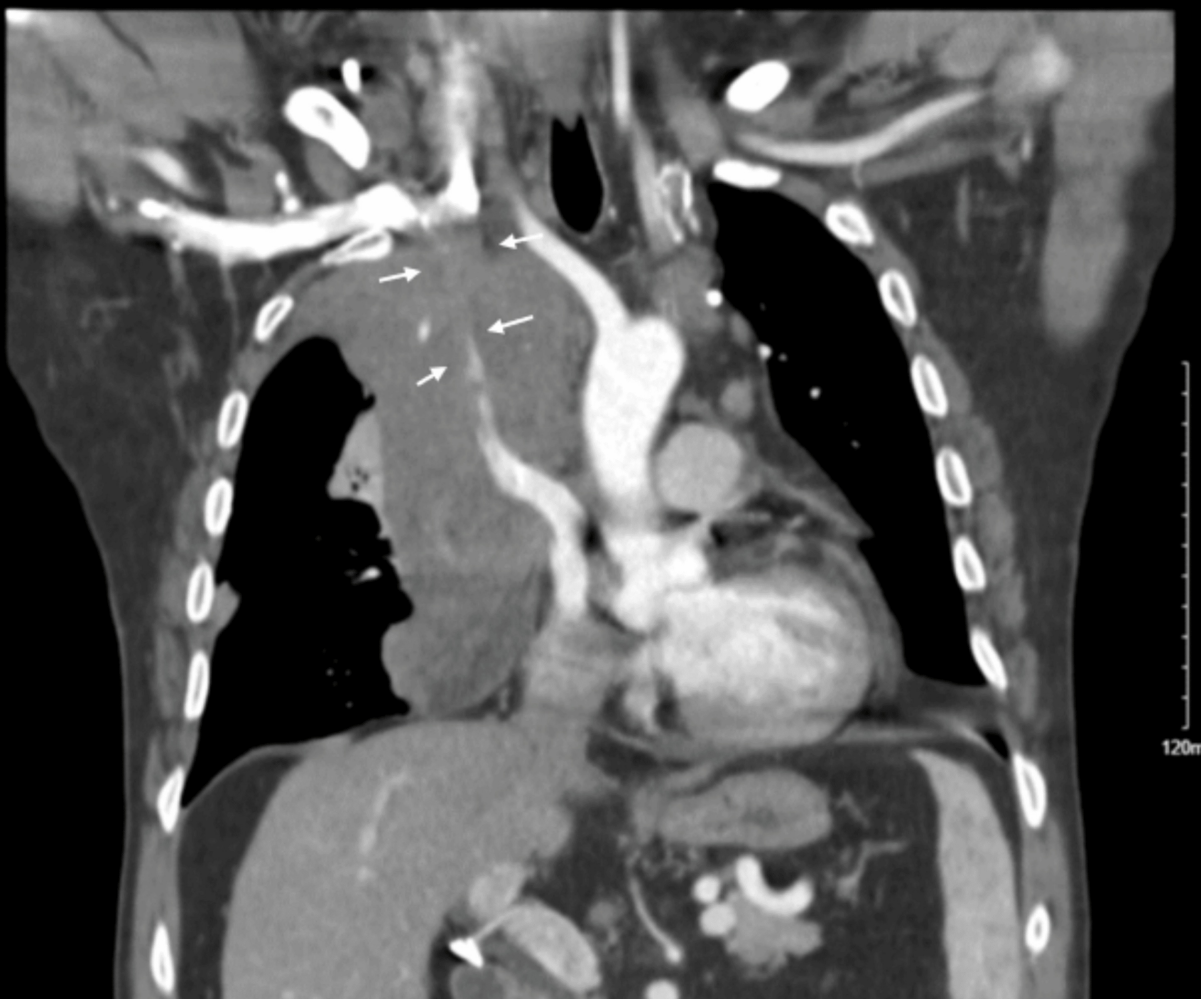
CT scan showing tumor compressing SVC causing compressive symptoms. CT: computed tomography; SVC: superior vena cava.
